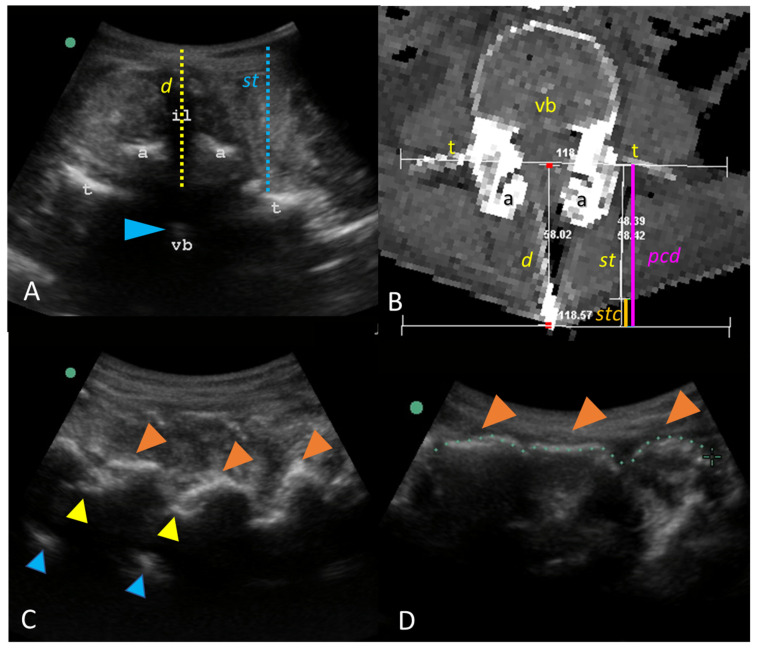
Ultrasound and CT measurements. (A) Axial view of the interspinous space. A slight hyperdense shadow corresponding to the vertebral body (vb)—arrowhead- is suggestive of the deepest level of the vertebral canal, delimitated between transverse (t) and articular (a) processes. The skin-to-dural sac distance (d)—discontinuous yellow line- may be determined across the dark shadow corresponding to the interspinous ligament (il). Note that it is comparable to the distance between the skin and the transverse process (st)—blue discontinuous line-. (B) Corresponding specular CT image of the same structures in an oblique view, perpendicular to the skin. The reduced (st) in thin patients was corrected by adding (stc)—orange segment- to obtain a parallel corrected distance (pcd)—magenta segment- comparable to the distance (d). (C) Best parasagittal oblique view. The deepest hyperechoic image (blue arrowhead) is suggestive of the level of the (vb) when the ultrasound can traverse the flavum ligament (yellow arrowhead) but not the spinous process (orange arrowhead). (D) Midline of the same case shows adjacent spinous processes, with a reduced interspinous space (common in older people) that precludes perpendicular needle insertion.
